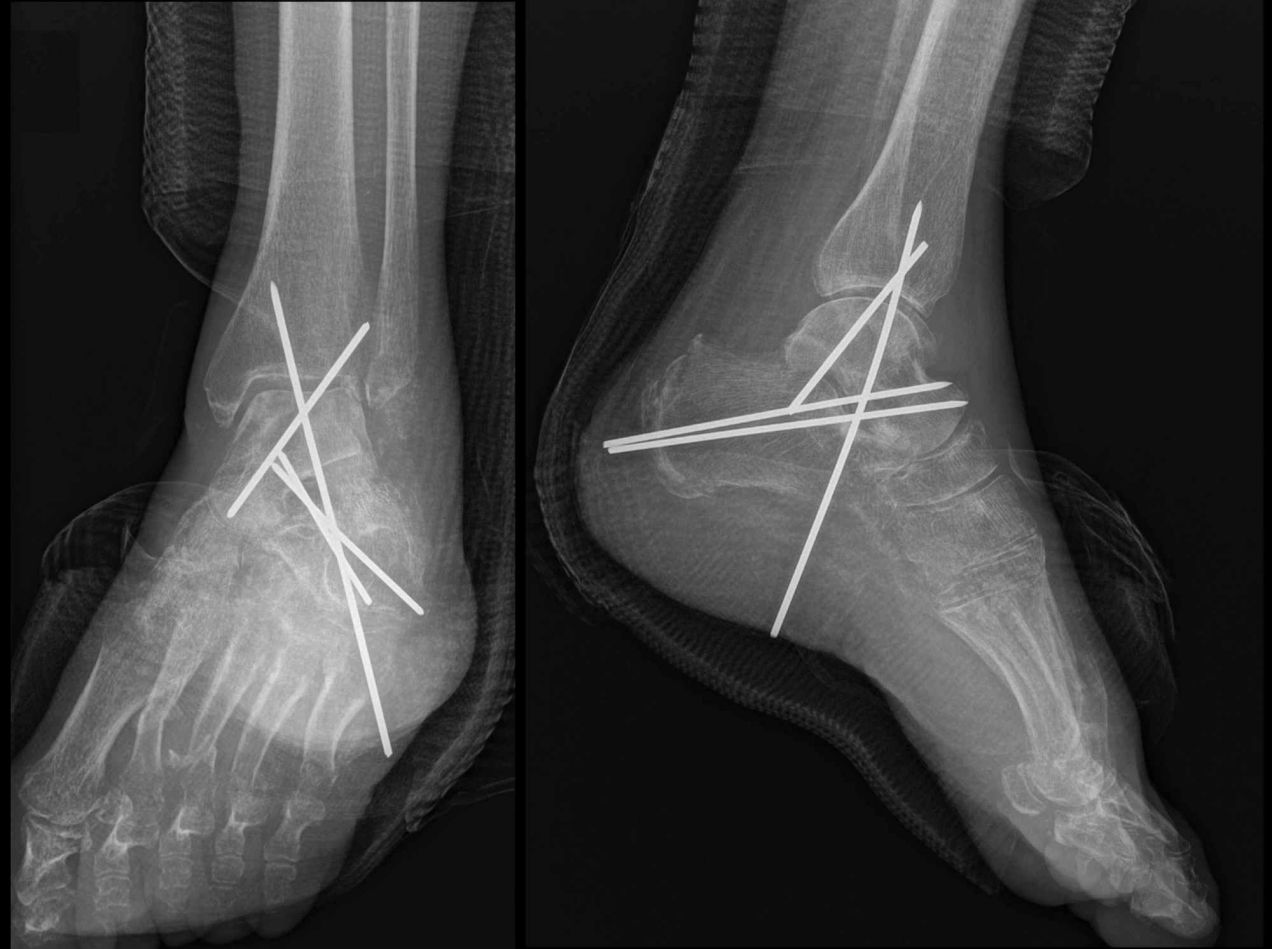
Radiography at three months showing an osteopenia of the ankle joints secondary to immobilization
